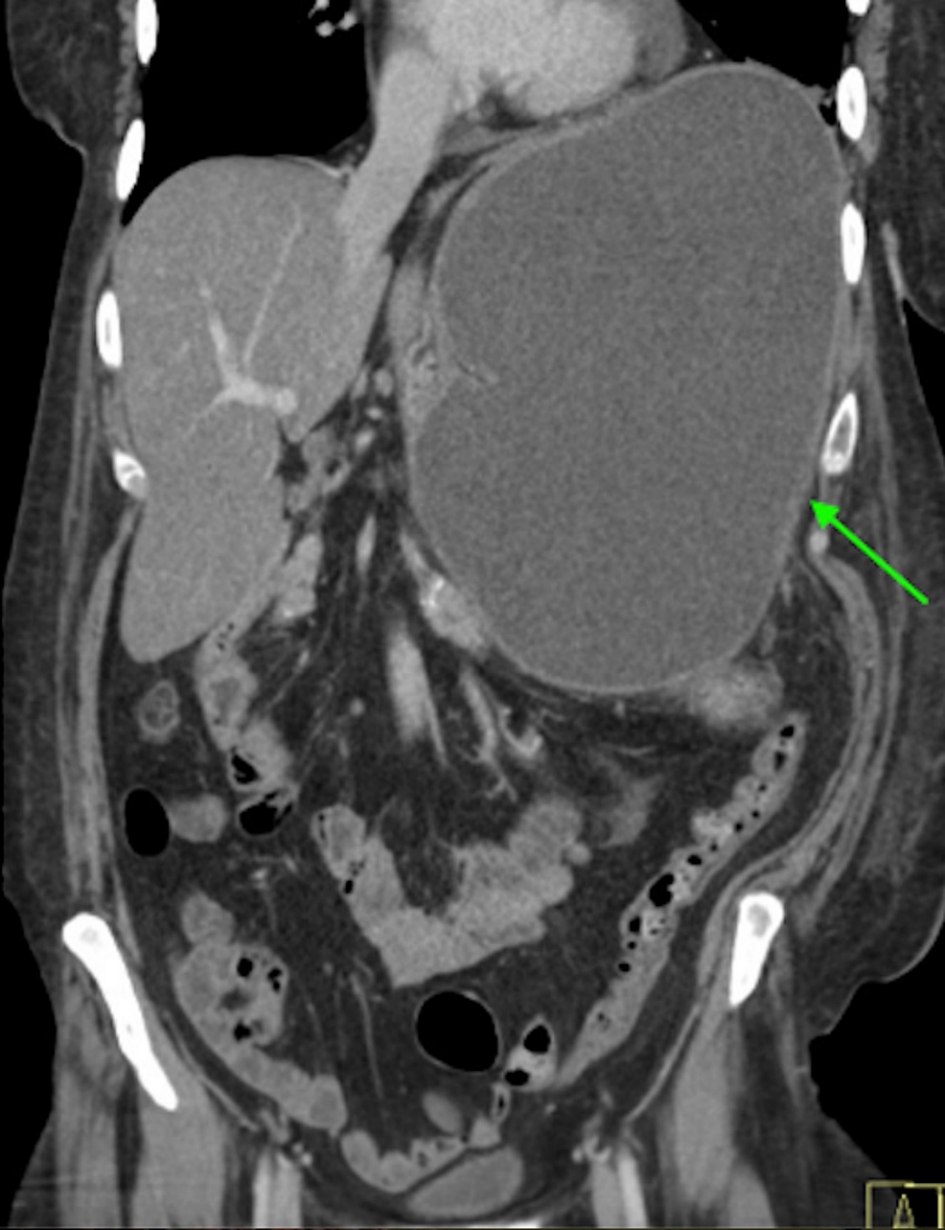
Coronal view CT of the abdomen and pelvis with intravenous contrast demonstrating a large fluid collection in the left upper quadrant (arrow). CT - computed tomography
